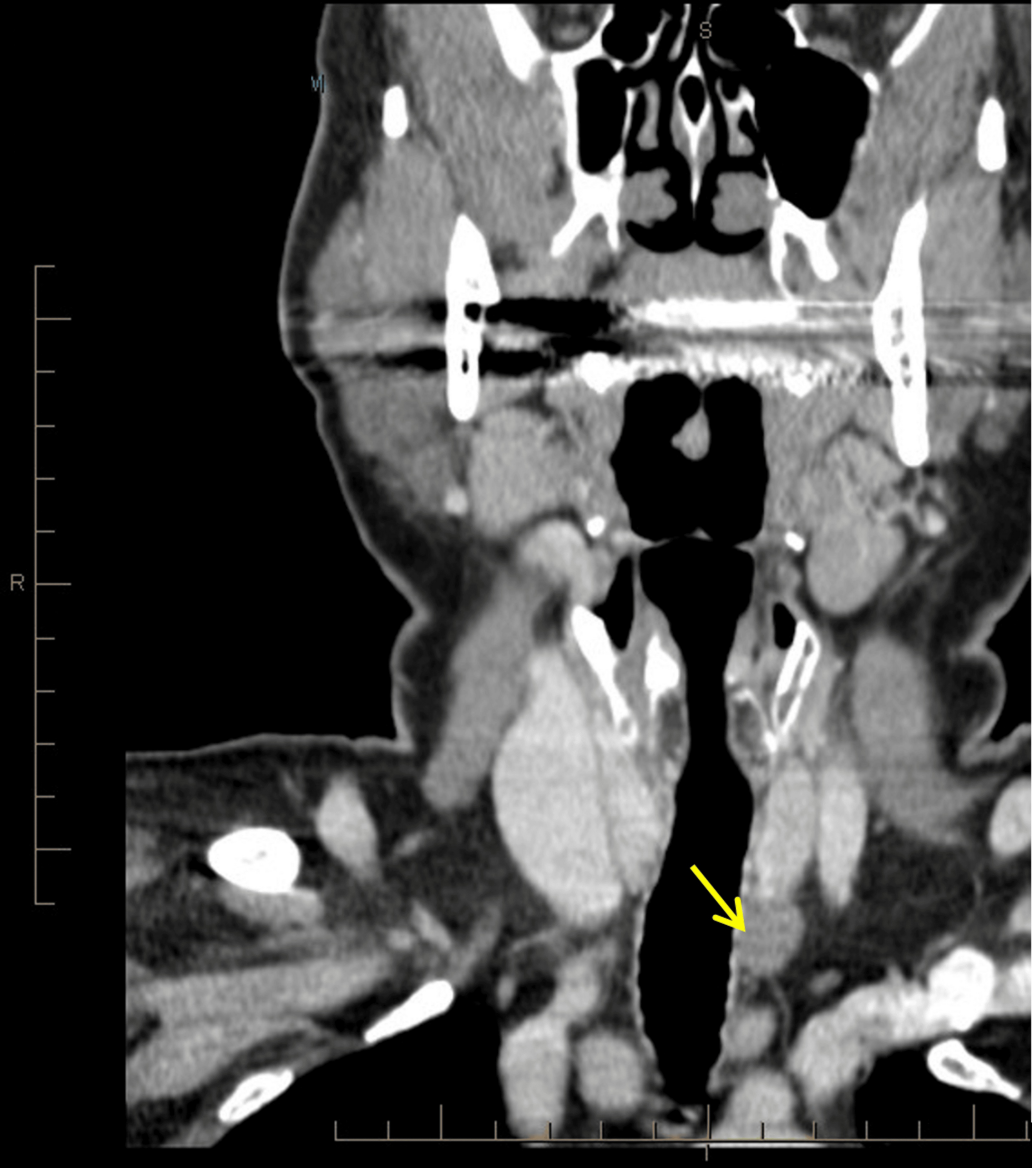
Follow-up neck CT scan with contrast. The yellow arrow indicates the schwannoma three years after the original scan.
